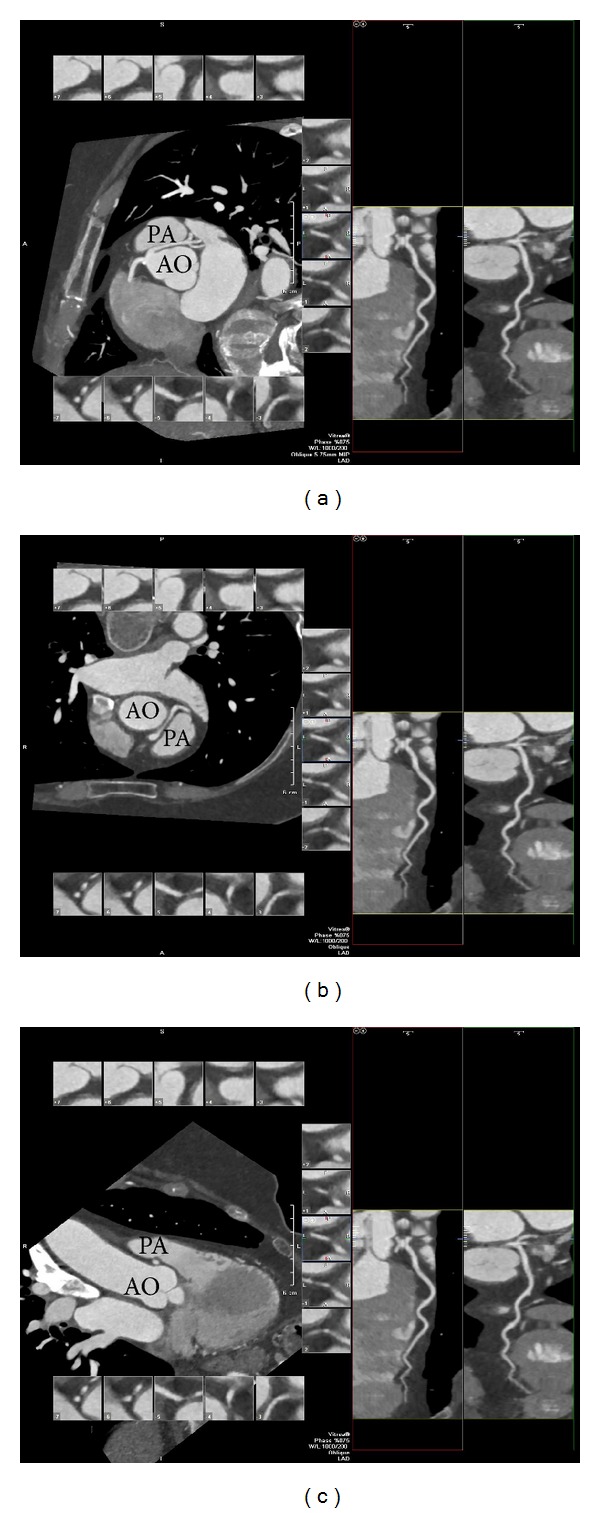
Cardiac computed tomography images of anomalous left main coronary artery off of the right sinus of Valsalva with a course between the pulmonary artery (PA) and aorta (AO).
